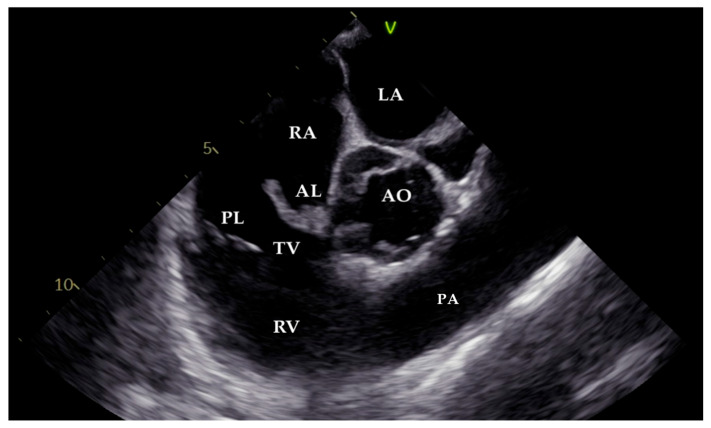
Transesophageal image (70°). Vegetation masses covering the anterior leaflet of the tricuspid valve. LA—left atrium; RA—right atrium; RV—right ventricle; AO—aorta; TV—tricuspid valve; AL—anterior leaflet; PL—posterior leaflet; PA—pulmonary artery.
